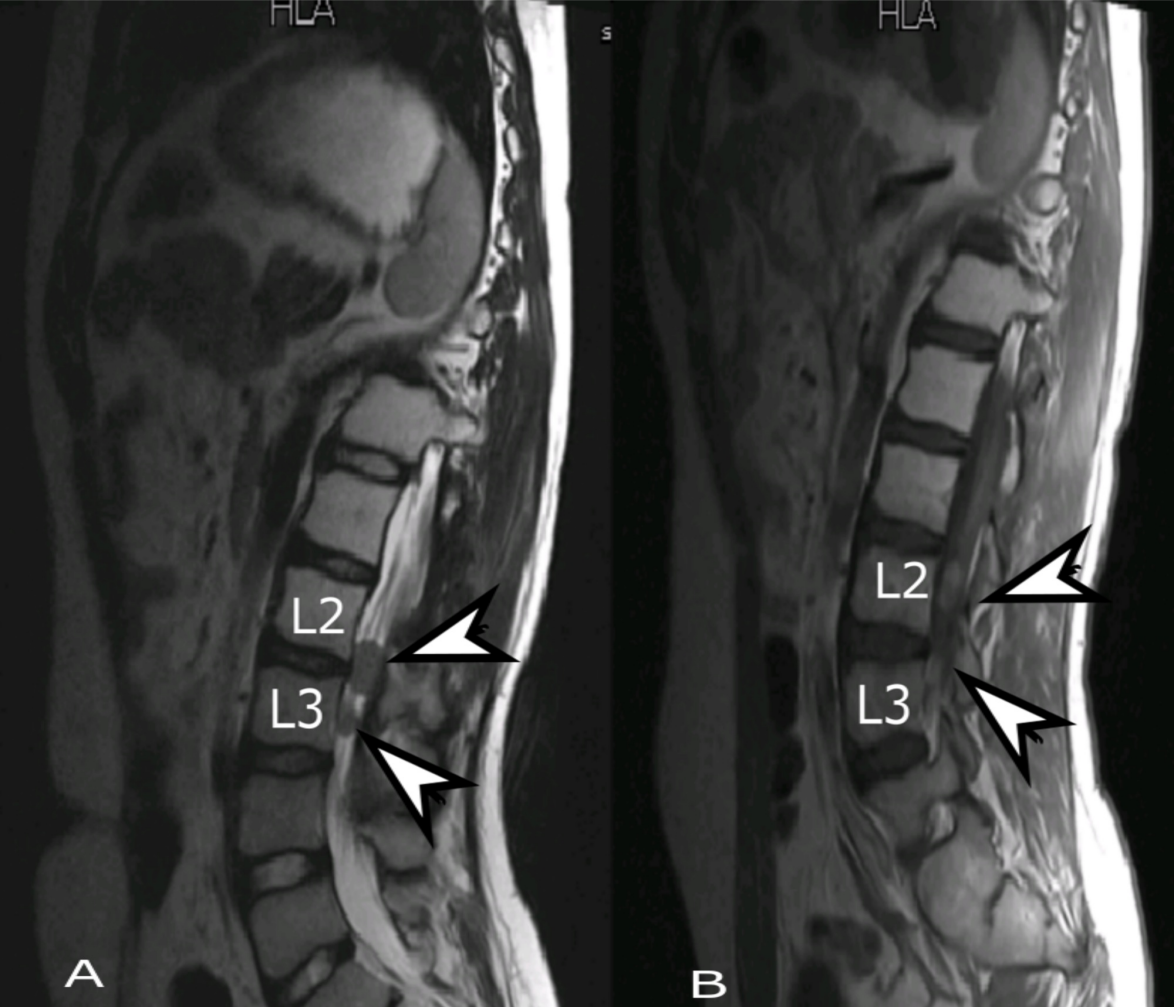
Follow-up MRI lumbar spine, sagittal view. (A) T2 weighted and (B) T1 weighted post-contrast images showing interval development of two new rounded lesions (arrows). MRI: Magnetic resonance imaging.
